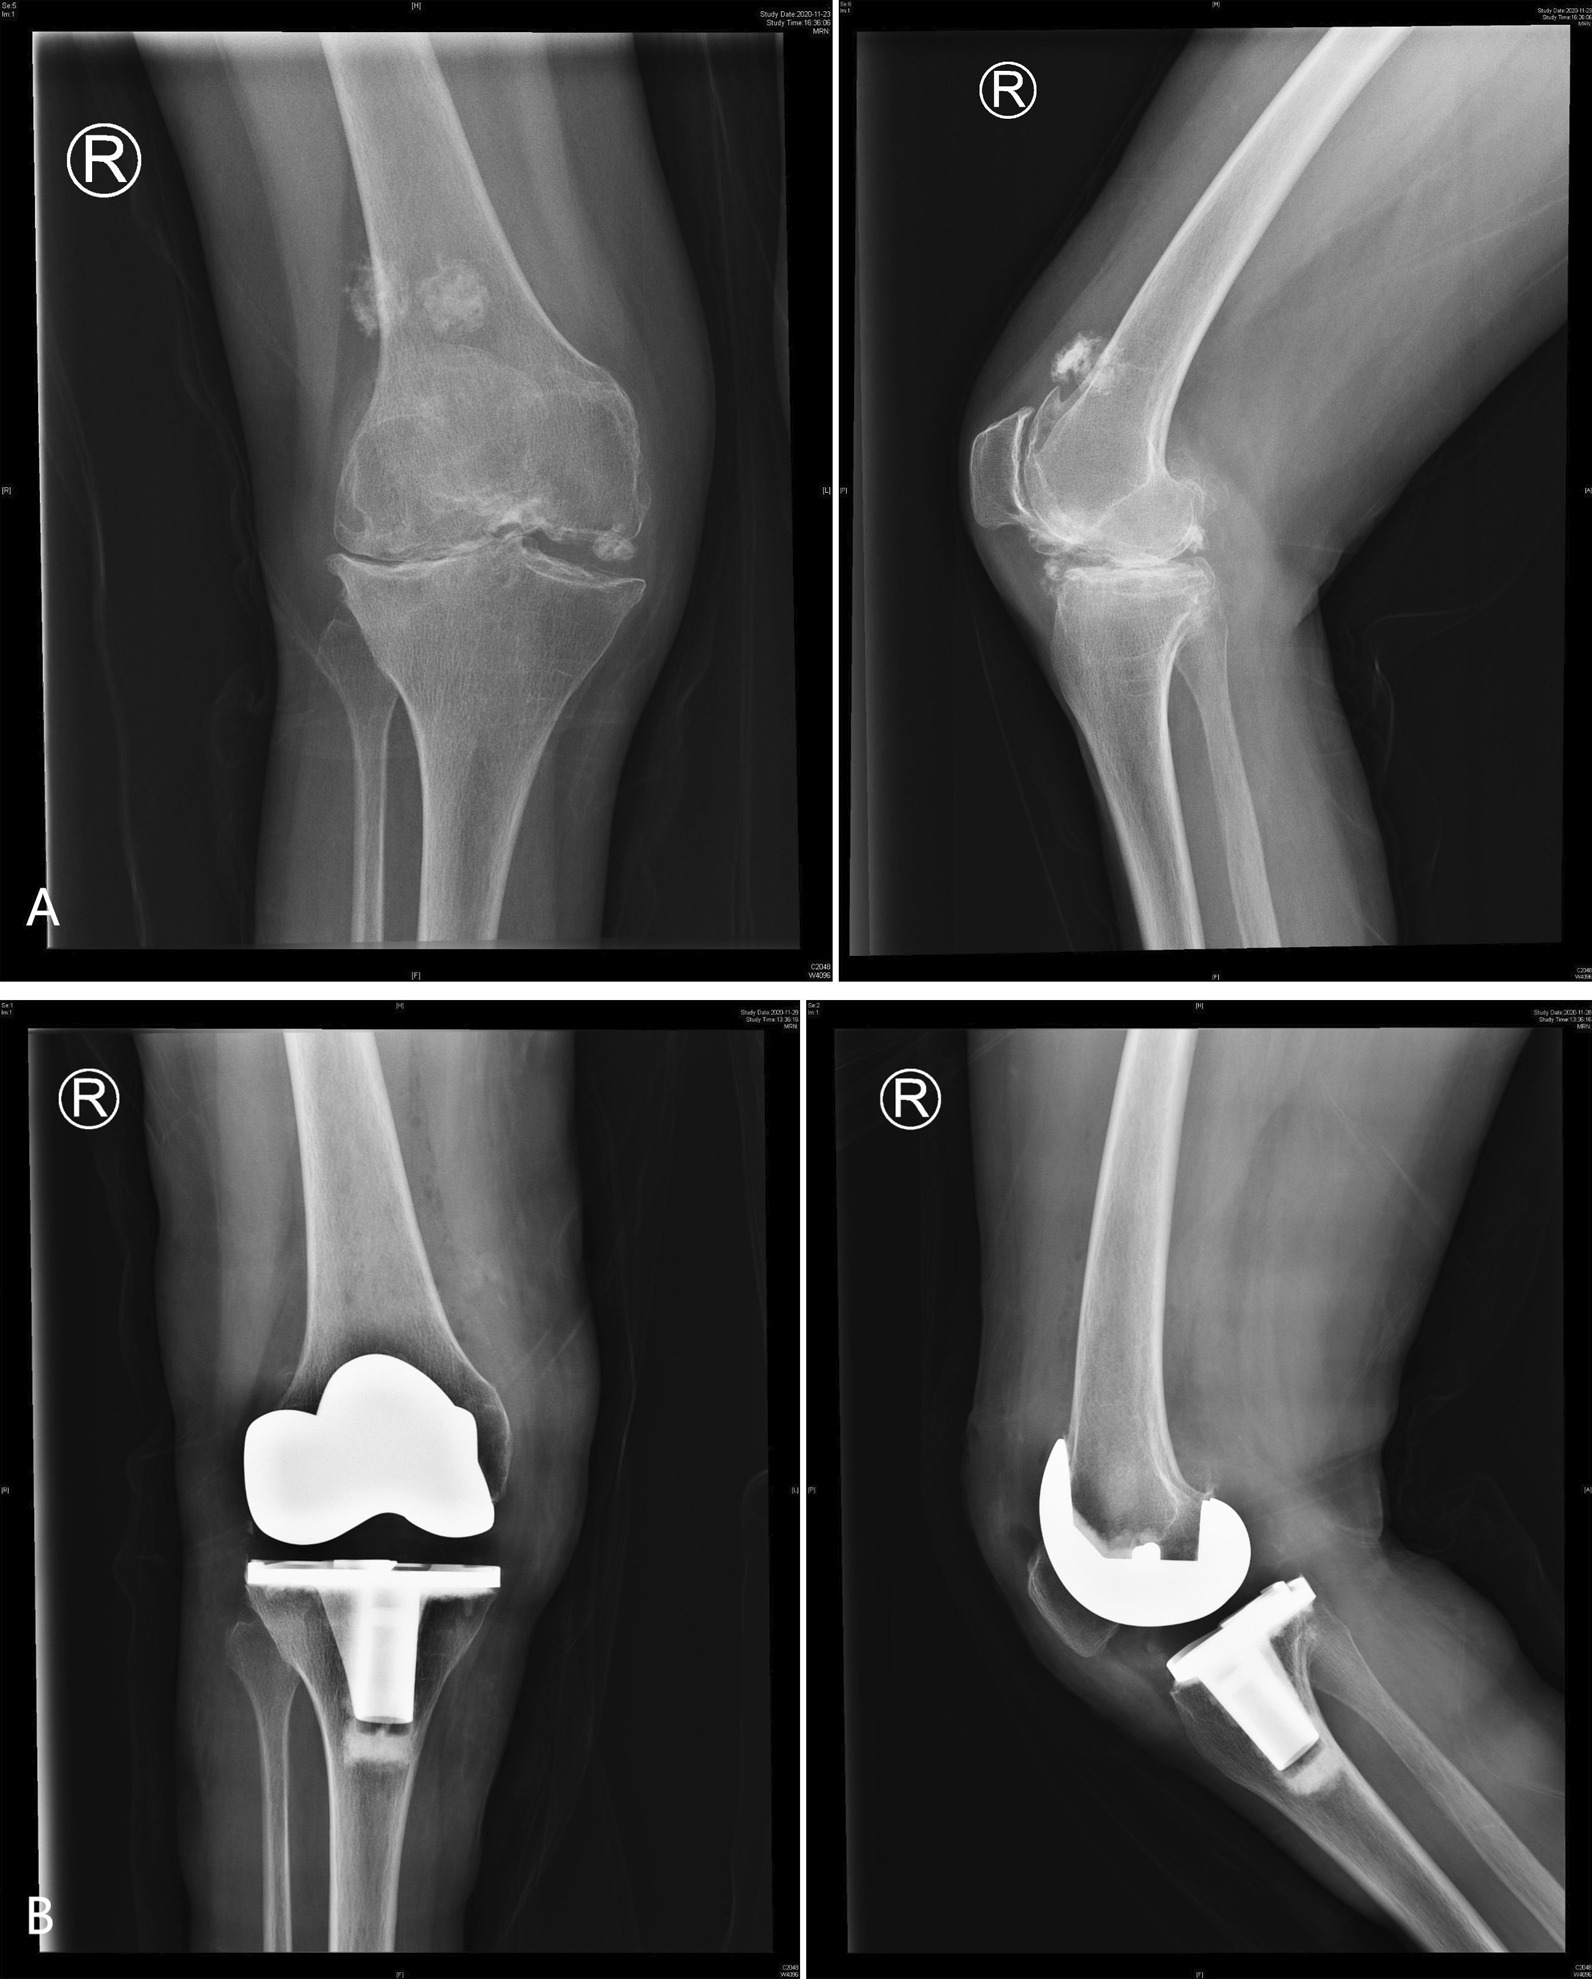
The anteroposterior and lateral knee radiographs of right knee before and after the operation. A preoperative valgus knee with osteophyte; B postoperative knee status
